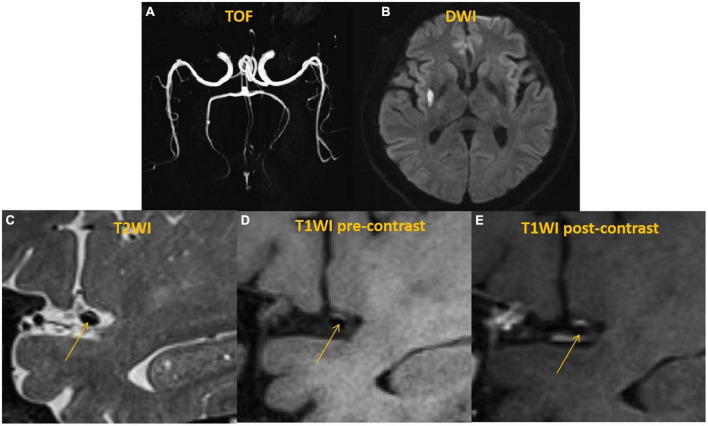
A 59 years-old man with acute ischemic stroke in the right basal ganglia. Time-of-flight (TOF)-magnetic resonance angiography (MRA) (A) demonstrated the stenosis located on the right middle cerebral artery (MCA). Diffusion weighted imaging (DWI) (B) showed a sheet acute infarcts in the right basal ganglia. High-resolution vessel wall MRI (hr-vw MRI) including T2-weighted image (C), pre-contrast T1-weighted image (D) and post-contrast T1-weighted image (E) visualized the plaque on the superior side of the right MCA. The plaque showed isointense to hyperintense on T2-weighted image (C) and pre-contrast T1-weighted image (D) and moderate enhancement on post-contrast T1-weighted image (E).
